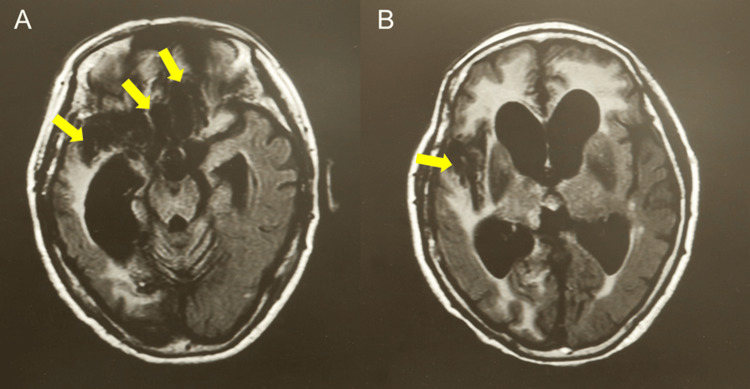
MRI FLAIR show cerebral contusions observed mainly in the bilateral frontal (A) and right temporal lobes (A and B). Yellow arrows indicate lesions of contusion. FLAIR: fluid attenuated inversion recovery
